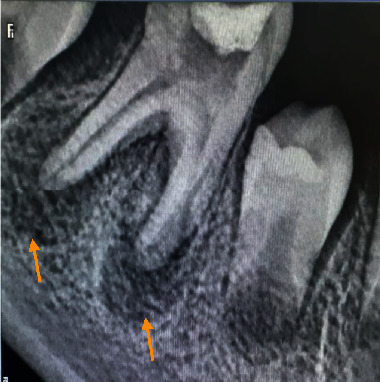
First mandibular molar with periapical lesions appended to the two root apices.
